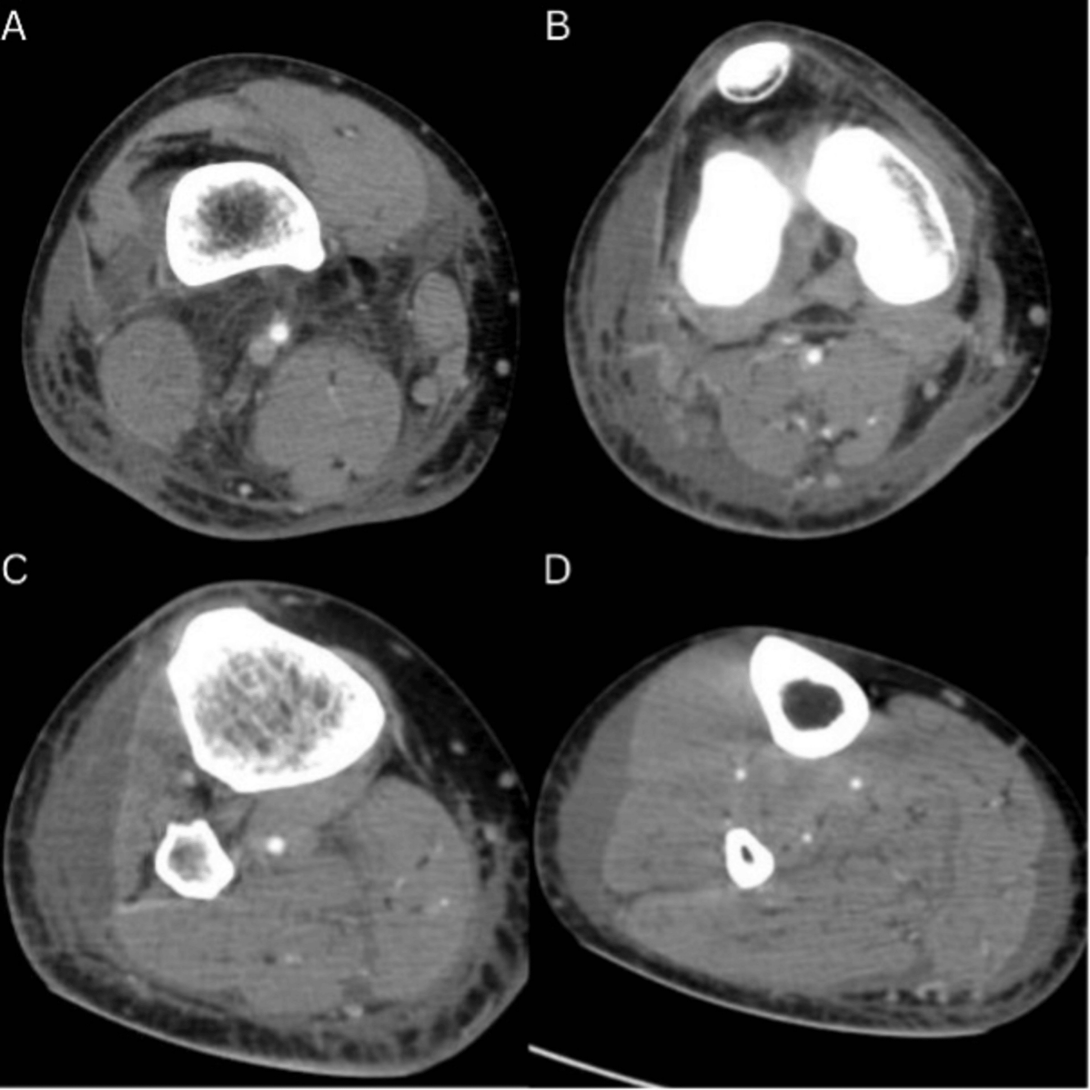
CT scan of the right leg at different levels (A-D) A) In the thigh, one can observe extensive oedema both at epifascial and subfascial levels. B-C) On an intermuscular plane of the hamstring muscles, the oedema can be observed in a concentric fashion as collections in front of the anterior compartment of the leg muscles; the oedema measures approximately 86 mm axially and is approximately 20 mm thick. D) Collection superficial to the distal third of the gastrocnemius with 51 mm of longitudinal width; the collection is parallel to the cutaneous surface.
